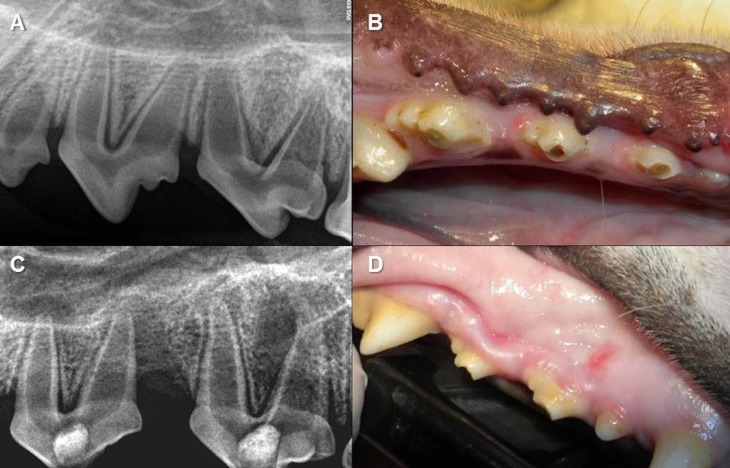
A) Initial periapical radiograph to confirm incomplete root formation and open apices; B) Root canals were left exposed to the oral cavity to allow microbial contamination; C) Periapical radiograph confirming apical periodontitis; D) Sinus fistula in relation with a tooth with chronic apical periodontitis.
